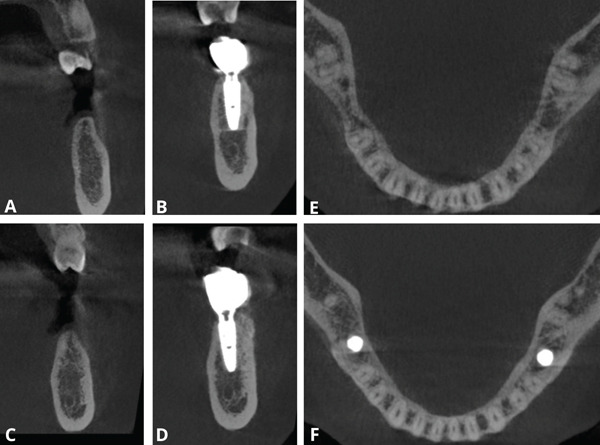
Comparative CBCT examination before and after implant treatment showing bone reconstruction at the augmentation site and correct implant positioning. (A) Cross‐sectional view before—Site 1. (B) Cross‐sectional view after—Site 1. (C) Cross‐sectional view before—Site 2. (D) Cross‐sectional view after—Site 2. (E) Axial views—before. (F) Axial views—before.
